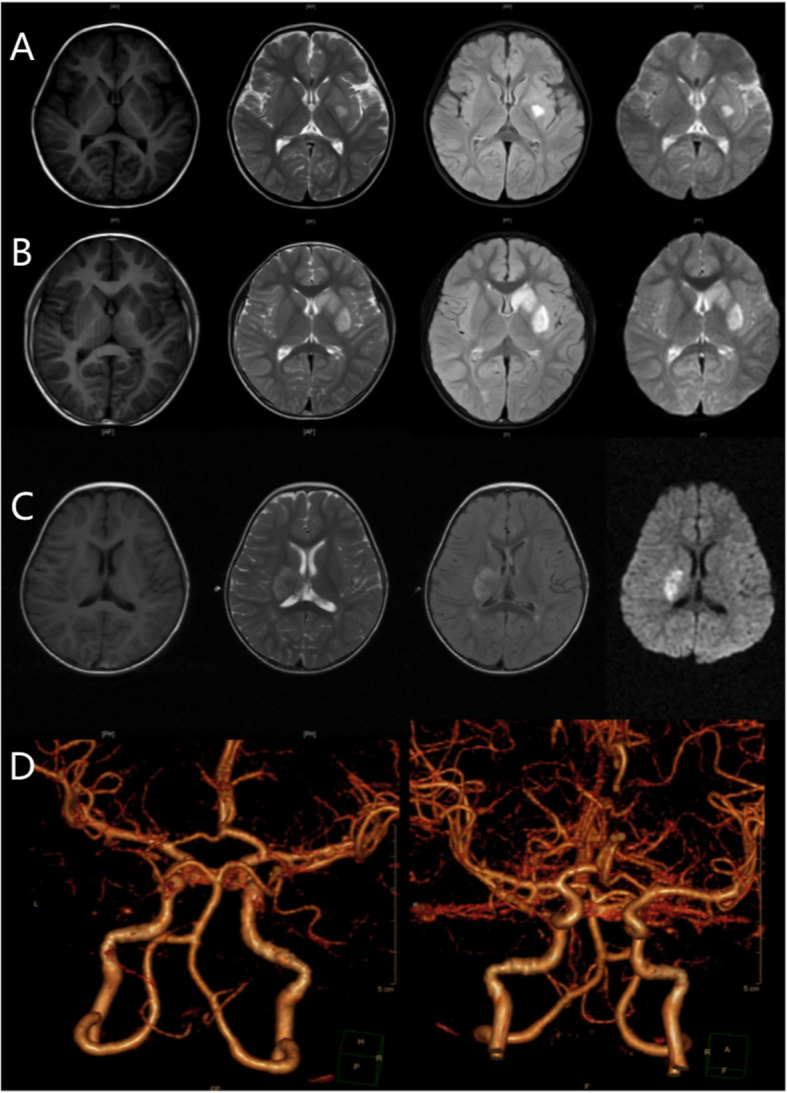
a Case 1, a 2-year-old boy presented with right facial paralysis accompanied by right limb fatigue for 48 h. The muscle strength of his right upper and lower limbs was grade II. The strength of his left limbs was normal. The right nasolabial groove was shallow, and the angle of his mouth was skewed to the left. MRI results showed that the left lenticular nucleus was involved. b Case 2, an 8-year-old boy had sudden hemiplegia of his right limb for 6 h without any inducement. His pronunciation was slurred. The muscle strength of his right upper and lower limbs was grade 0. The strength of his left limbs was normal. His right nasolabial groove was shallow. MRI results showed that the left lenticular nucleus with the caudate head was involved. c Case 3, a 1-year-old girl had left limb weakness for 48 h after falling from a height of 50 cm. The muscle strength of her left upper and lower limbs was grade III. Her left nasolabial groove became shallow, and the angle of her mouth was skewed to the right. The right lenticular nucleus with thalamus involvement was shown on MRI. The images from left to right were acquired by T1, T2, FLAIR and DWI. d The MRA of case 2 showed that the left anterior A1 segment was slightly thinner than the right anterior A1 segment, and the left lenticular artery was not clear
